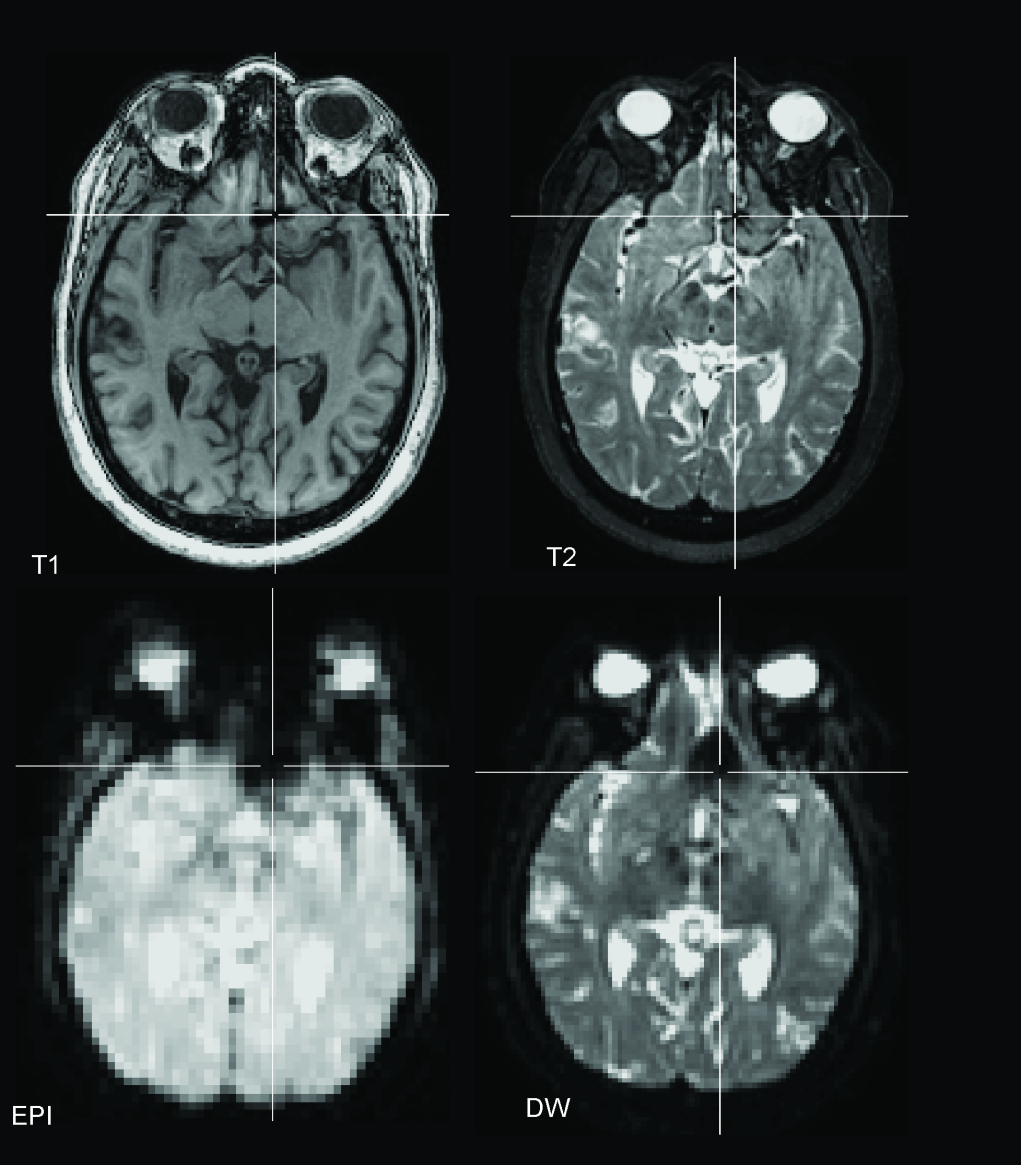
Appearance of Aneurysm Clip Artifact on Different Aligned MRI Sequences. Axial slices taken in the same imaging plane for Patient A are displayed in the axial plane (radiological convention) with the crosshair placed in the center of the clip artifact.
